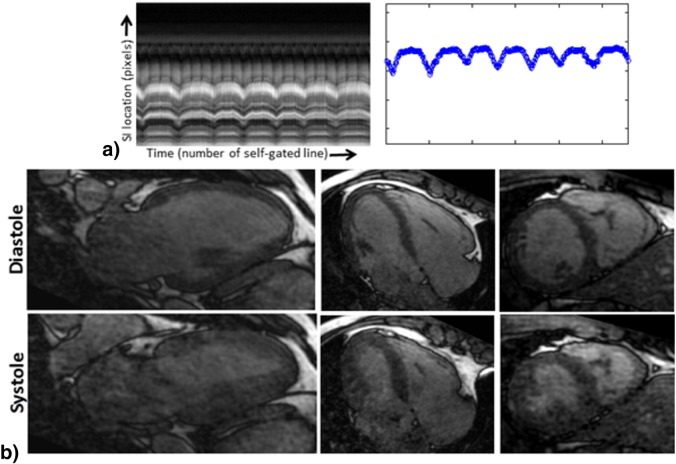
Self-gated 3D whole heart CINE MRI: a) 1D Superior-Inferior (SI) projection obtained from self-navigation in CASPR-Tiger trajectory and corresponding estimated 1D respiratory signal, b) from the reconstructed 4D volume, diastolic and systolic phases in two, four chamber and short axis orientations are shown for volunteer 1. Good quality reconstructions with isotropic resolution were observed in all orientations.
